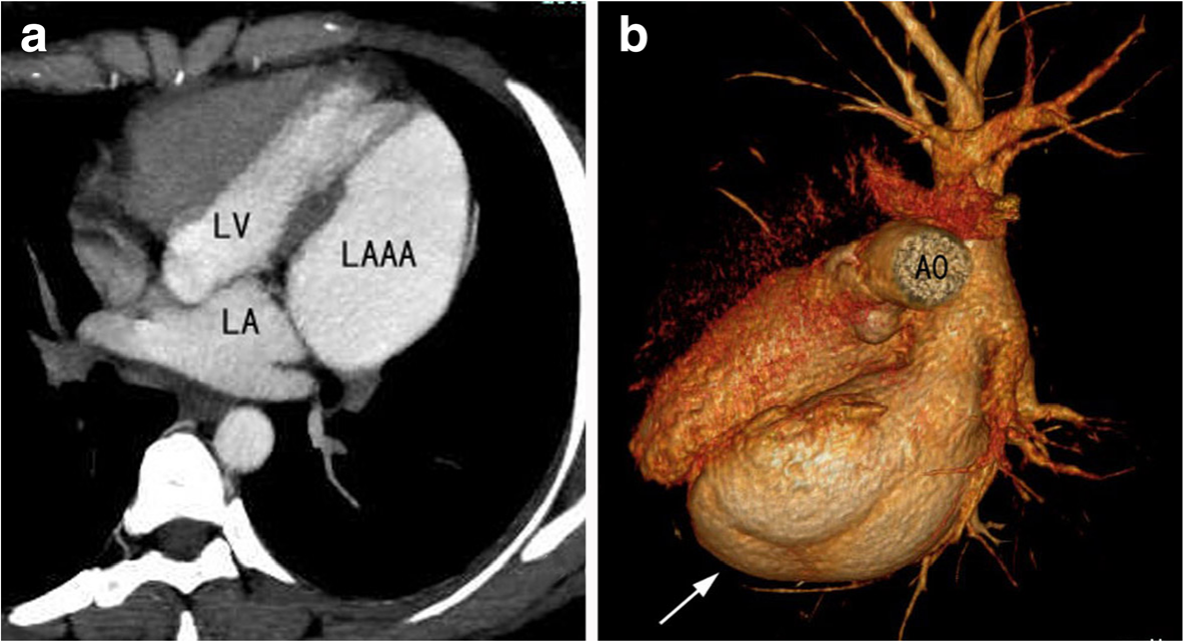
LAAA on CT images. a Thoracic CT showing the relationship between the appendage aneurysm and the left heart. b Three-dimensional CT image showing the spatial relationship between the LAAA (*arrow*) and the pulmonary veins and left heart. The LAAA was much larger than the LV
